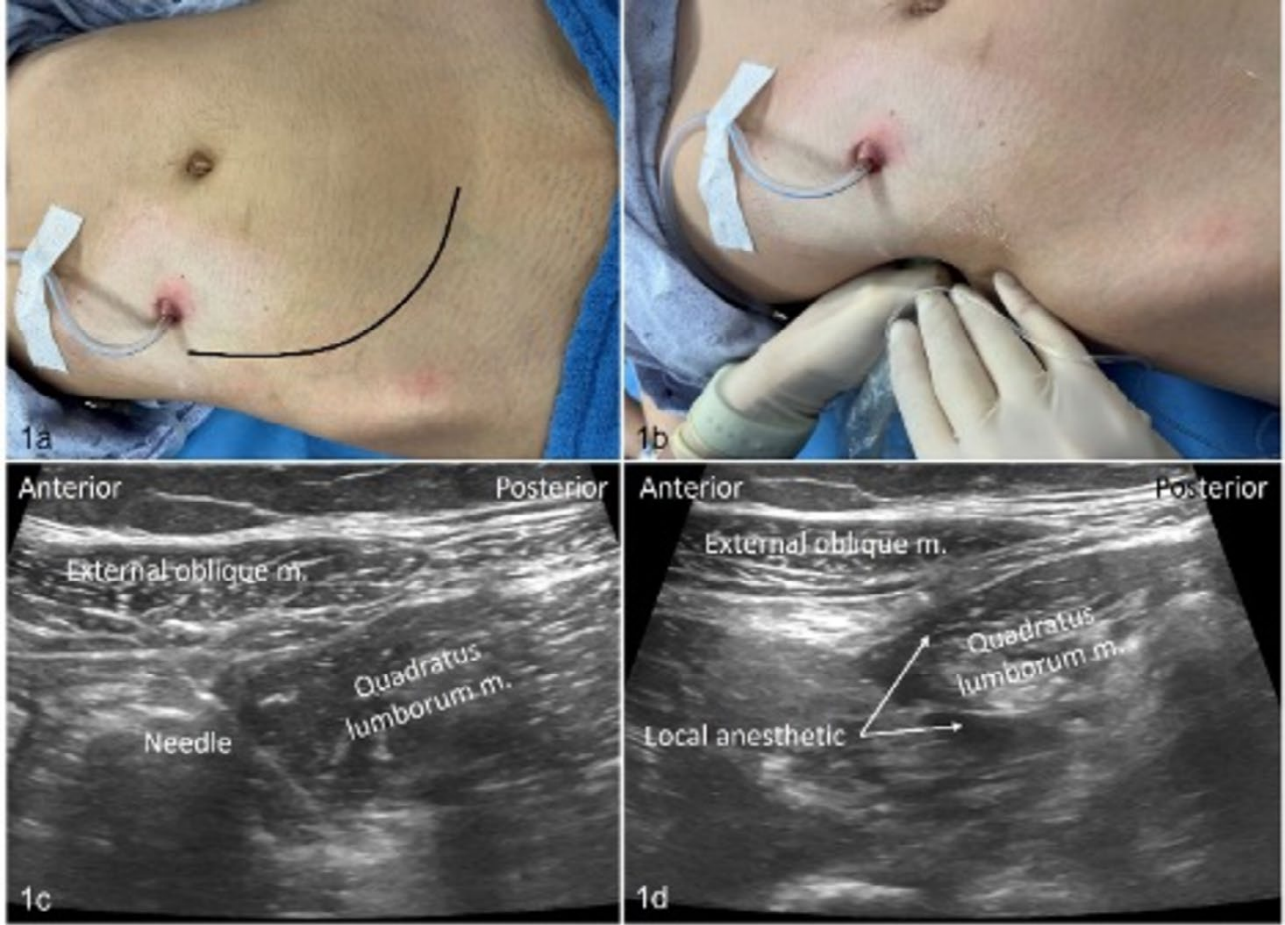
Quadratus lumborum block technique. (a) Incision location, (b) ultrasound probe and needle orientation, (c) ultrasound image of quadratus lumborum muscle and needle orientation, and (d) local anesthetic spread around the lateral and anterior borders of the quadratus lumborum muscle.
